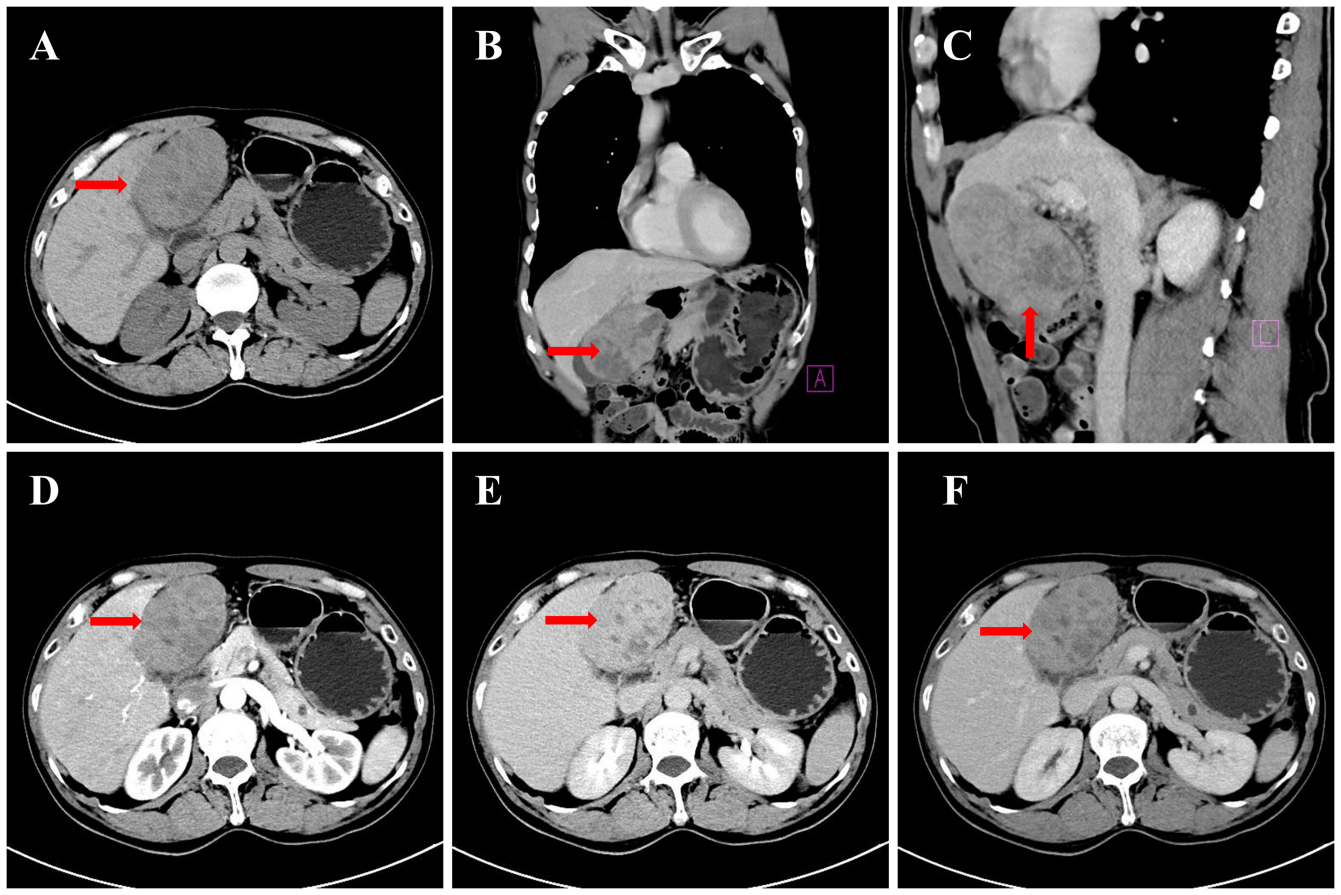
CT images of the upper abdomen: (A) Non-contrast axial view (left hepatic mass, arrow); (B) Non-contrast coronal reconstruction (mass compressing gallbladder, arrow); (C) Non-contrast sagittal reconstruction (mass-gastric antrum adhesion, arrow); (D) Contrast arterial phase (heterogeneous enhancement, arrow); (E) Contrast portal phase (parenchymal enhancement match, arrow); (F) Contrast delayed phase (capsular enhancement, arrow).
